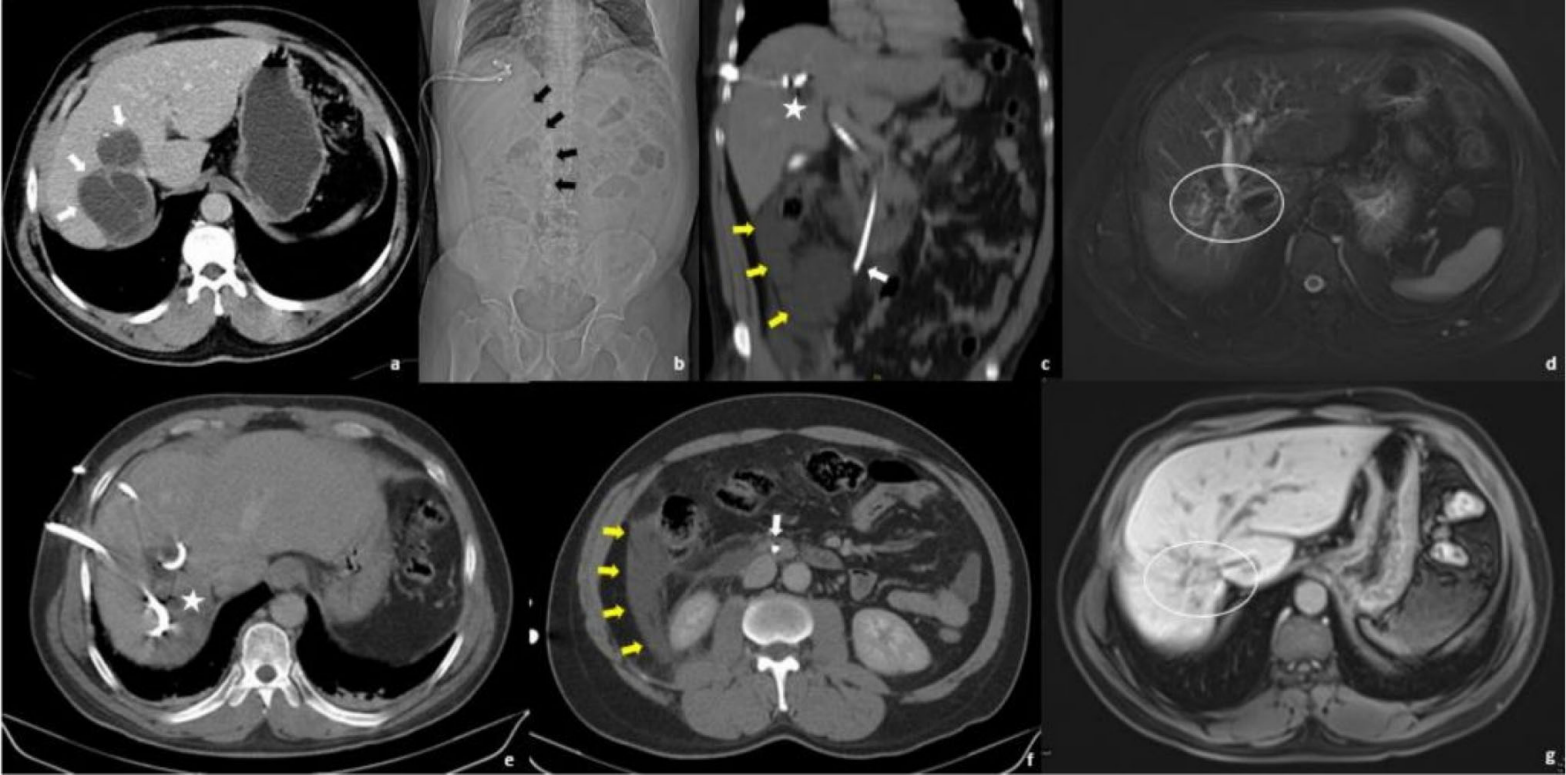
A 50-year-old male patient presented with bilobulated CE type 2 liver hydatid cyst (a, white arrows). During the procedure, cysto-biliary connection was detected at the cavitogram and the patient had bile-containing drainage from his catheter. ERCP was performed 5 days after the procedure, and a biliary stent was inserted. After 20 days, the patient presented with acute abdominal pain to the emergency room. It was obvious that some air bubbles (c and e, asterisk) within the cyst consistent with infection and lower tip of the choledochal catheter pass through the duodenal wall and extent to the right retroperitoneum (c and f, white arrows; b, black arrows); also there is some collection in there (c and f, yellow arrows). The ERCP catheter was removed, a catheter was inserted into the collection on the same day, and all these catheters were removed 5 weeks later when drainages were less than 5 ml per day. Two years later, control MRI revealed that there is no cyst or infection at these sites but some sequelae changes (d and g, white circles)
